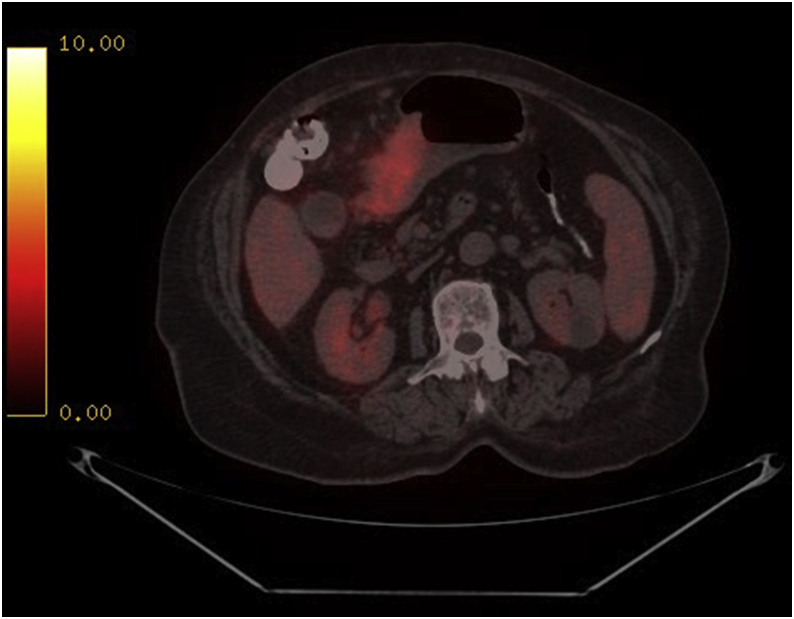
PET-CT scan pre-operatively showing focal FDG avidity in the antro-pyloric region suggestive of malignant mass.
